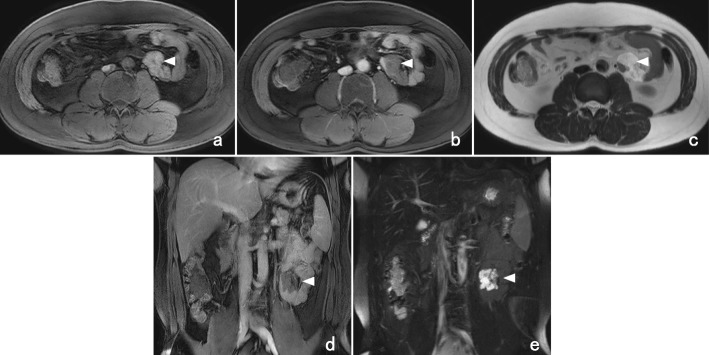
MRI findings. a MRI showed the mass with intermediate intensity on T1WI (white arrowhead). b The enhanced phase on T1WI showed the mass with a relatively low intensity (white arrowhead). c On T2WI, the mass was depicted as a high-intensity tumor (white arrowhead). d Coronal view on T1WI. The irregularly shaped mass with a low signal intensity compressed the adjacent jejunum (white arrowhead). e Coronal view on T2WI. An accumulation of cystic lesions with a variety of sizes was more clearly depicted (white arrowhead)
